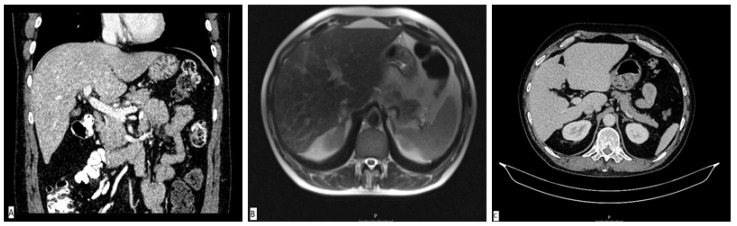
Radiological imaging of the liver parenchyma. MSCT scan of liver performed in March 2017 showing normal liver parenchyma (A). MR scan of liver performed in October 2017 showing a rough structure of the right liver lobe with initial retraction of the liver parenchyma, suggesting initial cirrhotic changes (B). Liver CT scan from March 2019 demonstrating liver cirrhosis (C).
